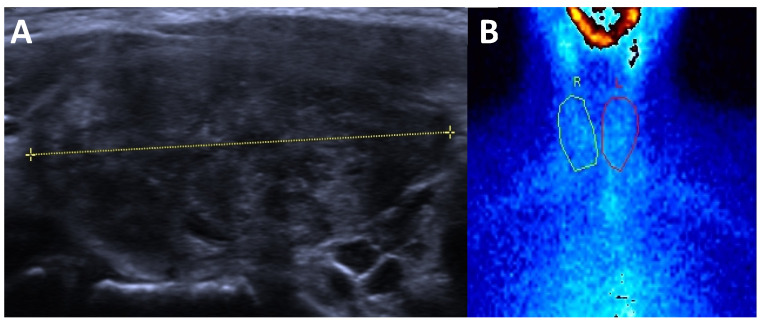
Subacute thyroiditis (A) ultrasound: enlarged thyroid that is markedly hypoechoic and hererogeneous and (B) ^99m^Tc-pertechnetate scintigraphy: absent thyroid activity.
